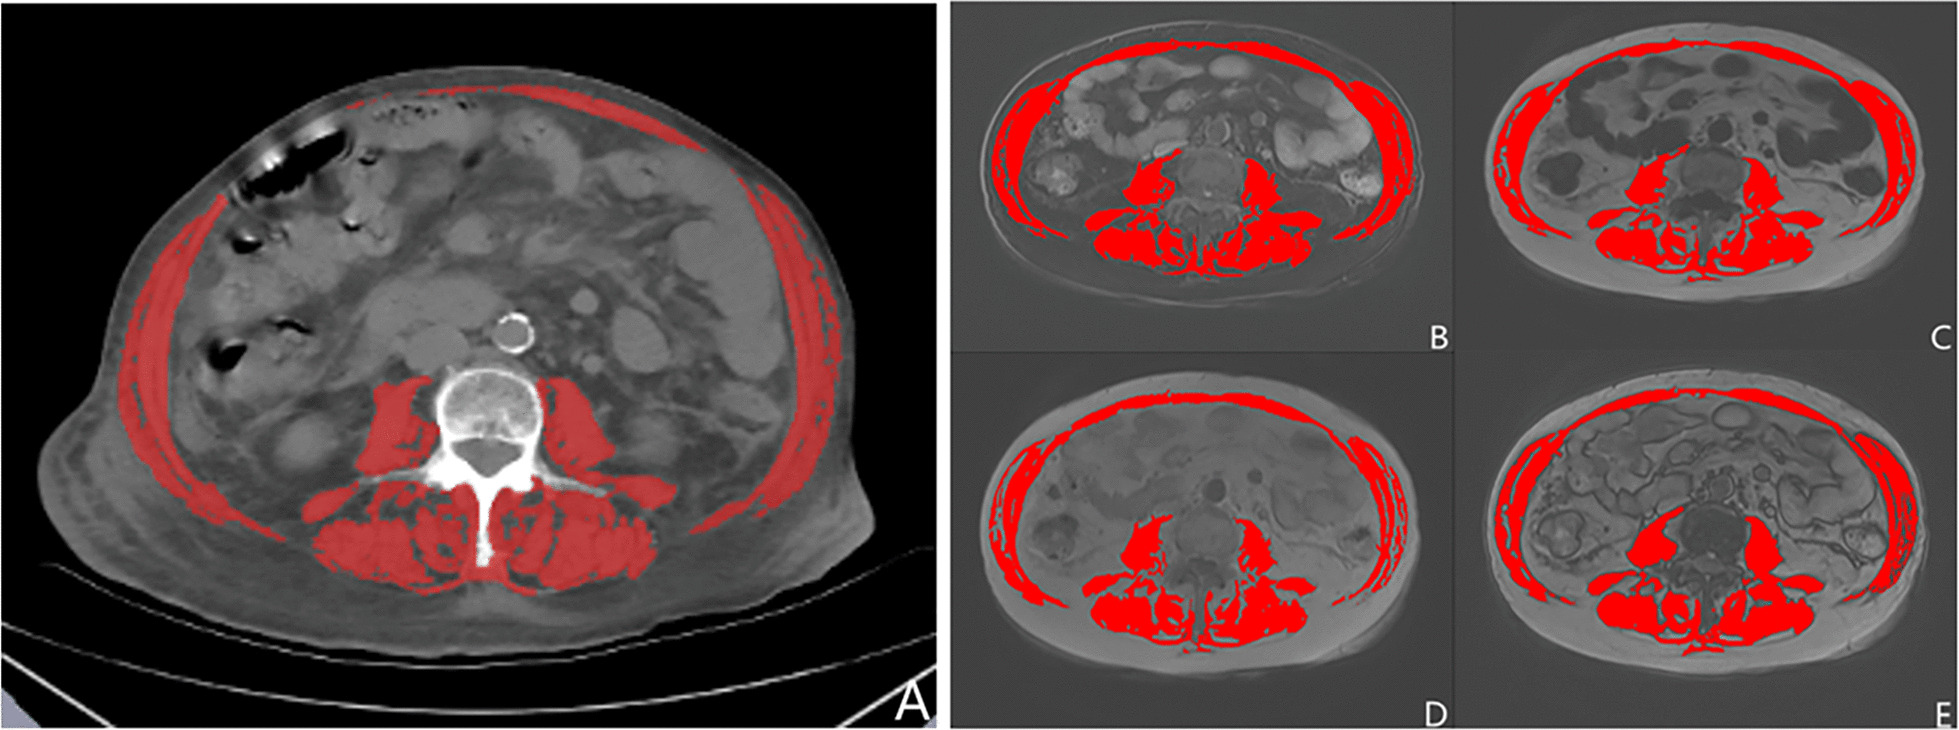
Skeletal muscle area segmentations from CT and MRI. A CT images, B T1-weighted (T1w) water images, C T1w fat images, D T1w in-phase images, and E T1w out-of-phase images
